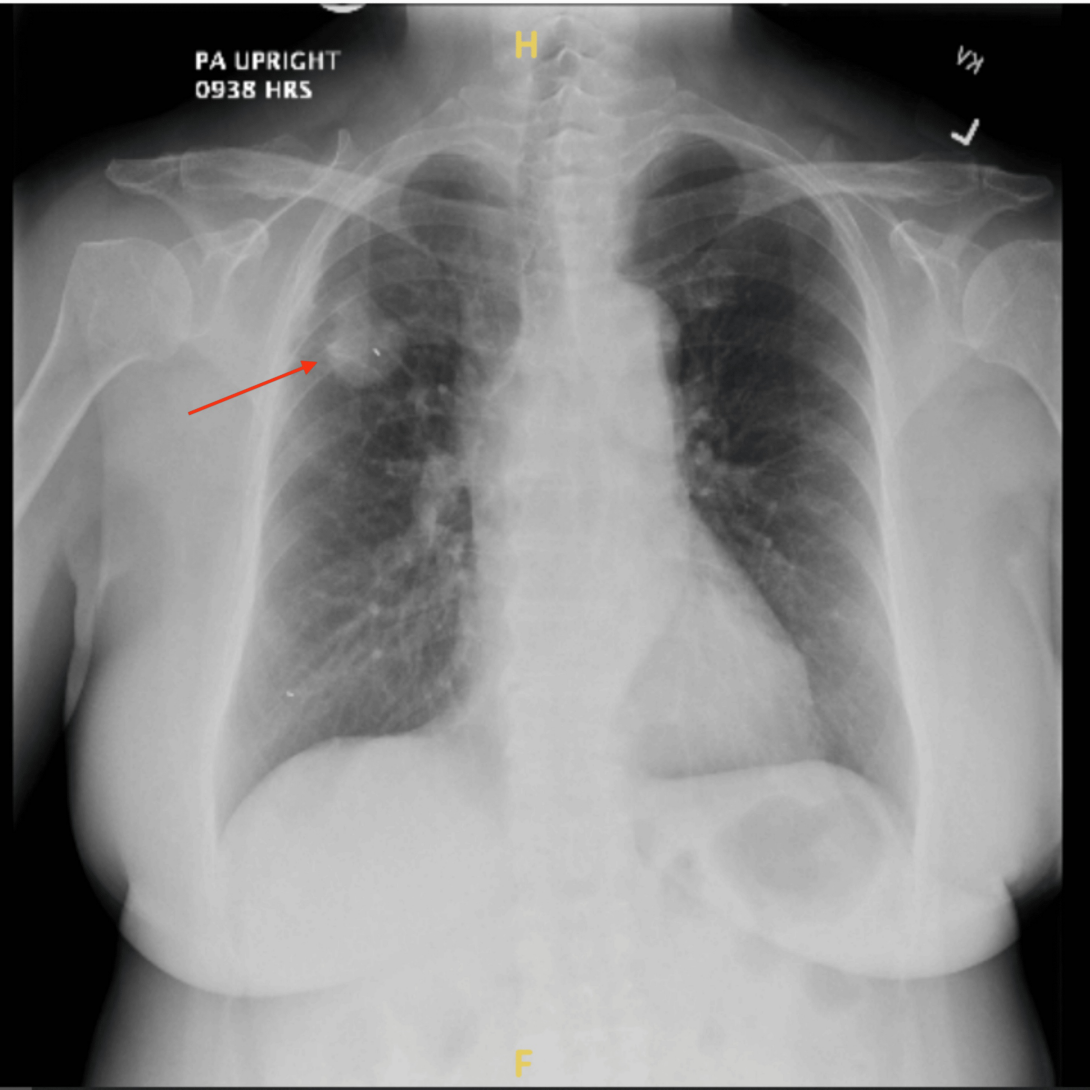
Right upper lobe mass identified on chest X-ray The arrow shows the right upper lobe mass. Ground glass nodules in the left and right lower lobes are better visualized on CT, which is unavailable CT: computed tomography
